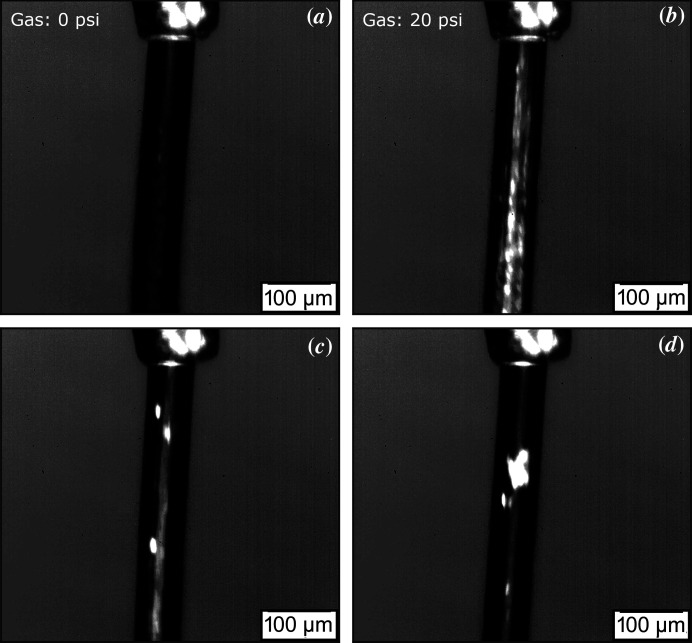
Images of the sample stream taken in air with the sample between crossed polarisers. Bright regions of the images indicate the presence of the polarizing lamellar phase. The sample composition is 60:40 MO/water (*w*/*v*) and the nozzle diameter is 75 µm. (*a*) Stabilizing gas sheath turned off and sample is in a non-polarizing cubic phase. (*b*) Gas sheath on with backing pressure of approximately 20 psi. Brightness of the sample indicates the presence of lamellar phase. (*c*, *d*) The appearance of isolated lamellar regions not associated with changes in gas pressure. These are likely caused by air bubbles within the sample and are consistent with the transient appearance of L_α_ observed in the X-ray data.
